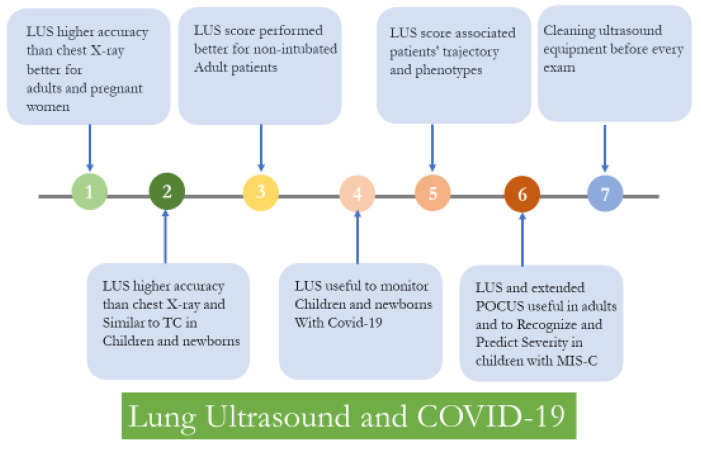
Main steps in the implementation of lung ultrasound in patients with COVID-19.
